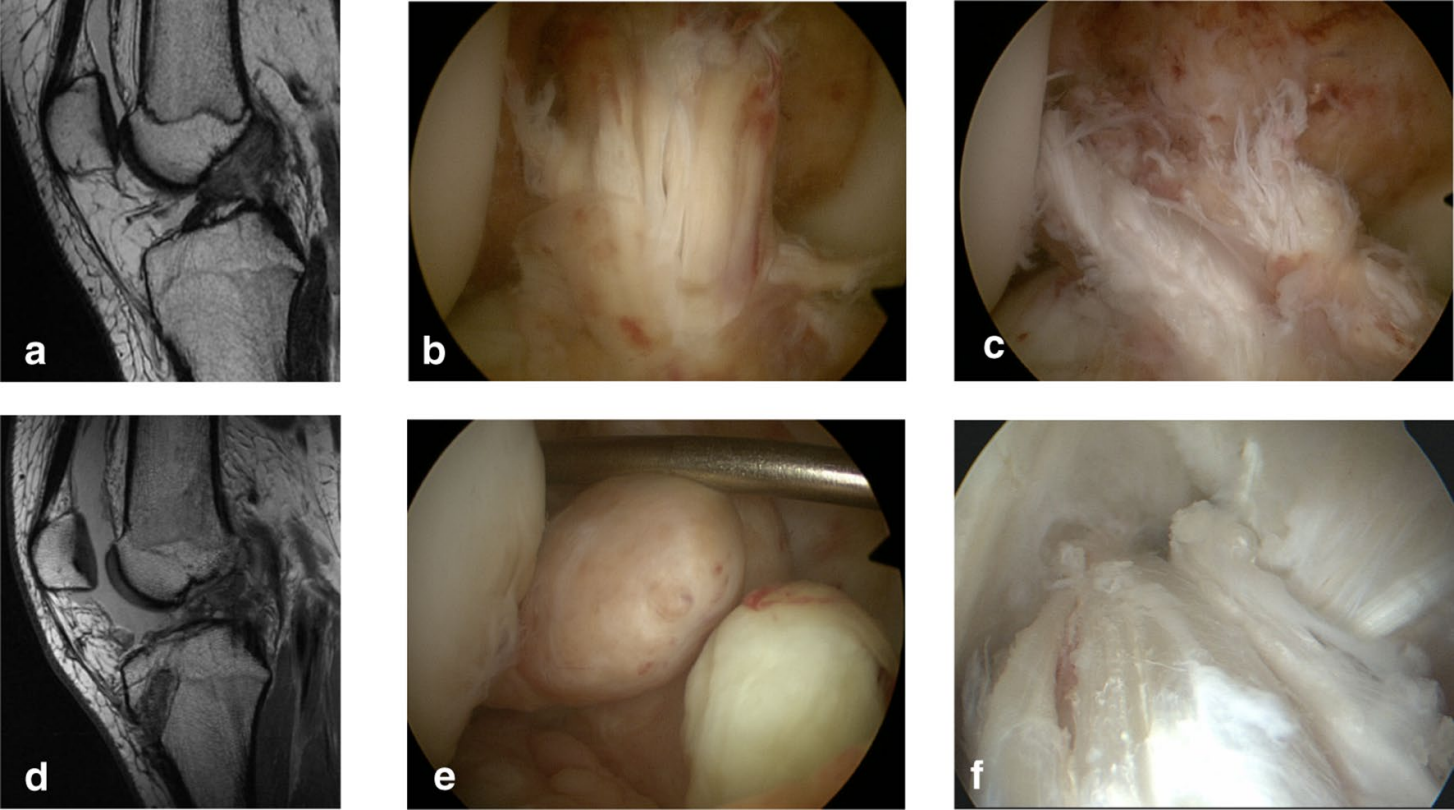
a MRI view of ACL rupture, b arthroscopic view of the ACL tear, c arthroscopic view DIS repair, d MRI view of the non-healed ACL repair, e arthroscopic view of the non-healed ACL, f arthroscopic view of the notch after autograft ACL reconstruction
